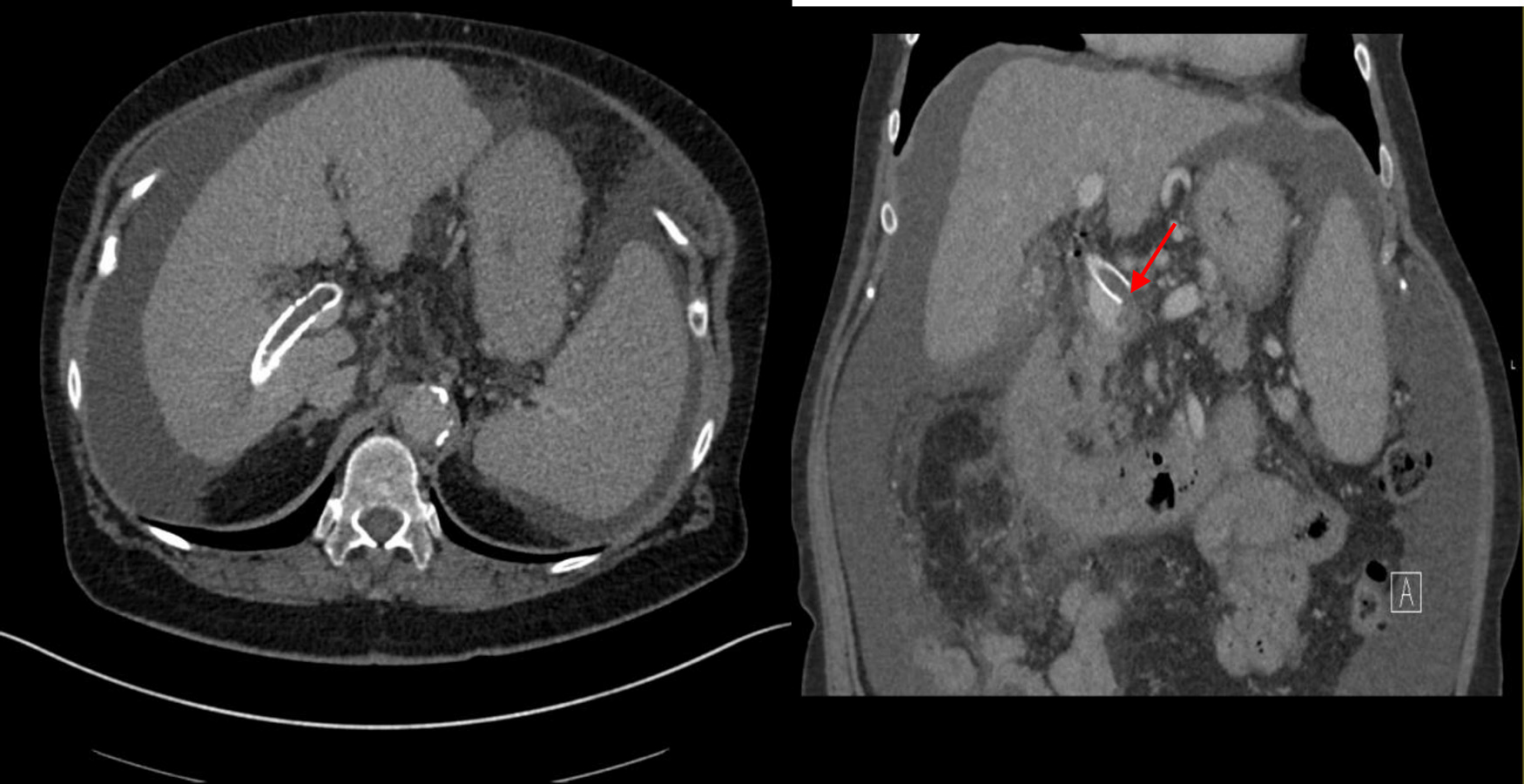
Venous phase of a contrast-enhanced CT of the abdomen showing thrombus within the TIPS stent extending to the superior mesenteric vein inflow. Both axial (Fig. 3A) and coronal images (Fig. 3B) are shown.
